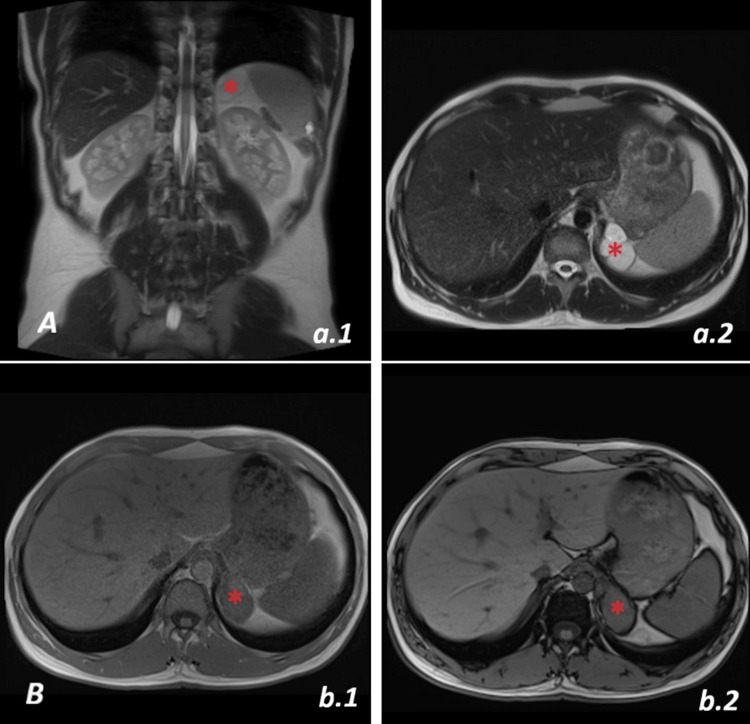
Thoracic magnetic resonance Imagering (MRI) A lesion showing high signal intensity on T2-weighted images and low signal intensity on T1-weighted images, without a fatty component was observed in the left adrenal compartment. A. Bronchogenic cyst (*) on MRI with high intensity on T2 weighted images a.1: In coronal plane; a.2: In axial plane B. Bronchogenic cyst (*) on MRI with low intensity on T1 weighted images (axial view) b.1: In-phase T1-weighted MRI image; b.2: Out-of-phase T1-weighted MR image
